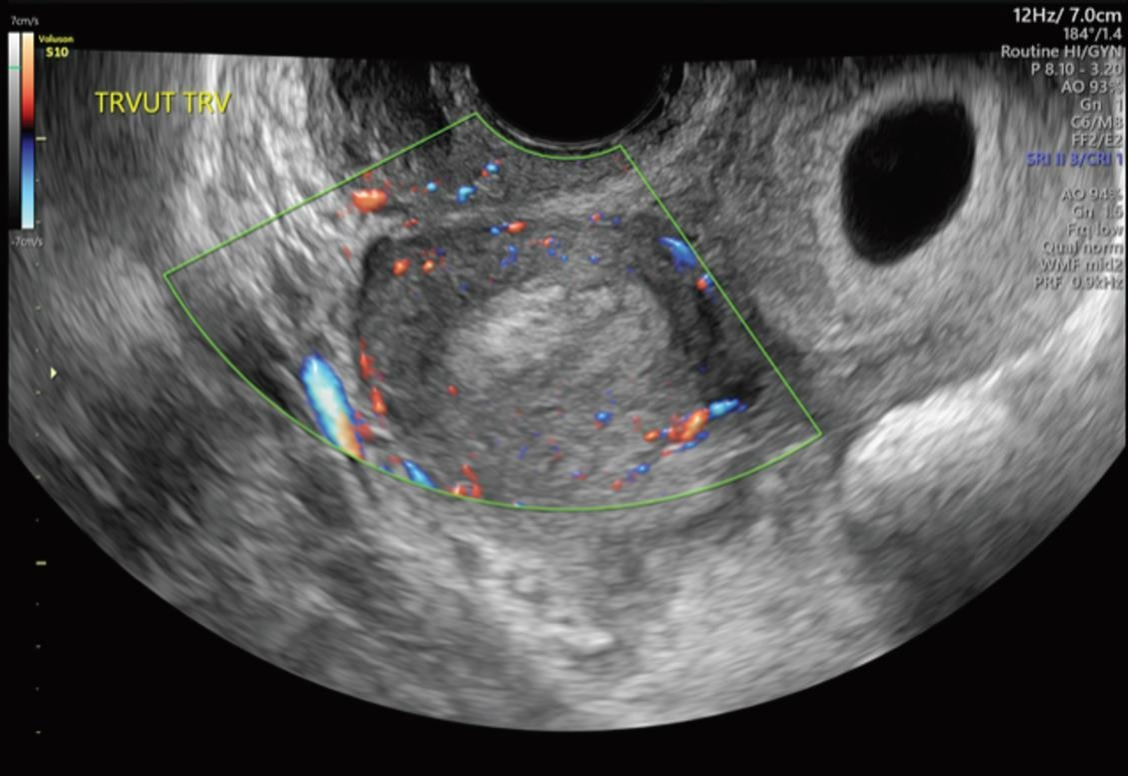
Transvaginal ultrasound demonstrating heterogenous material in right uterine horn.
